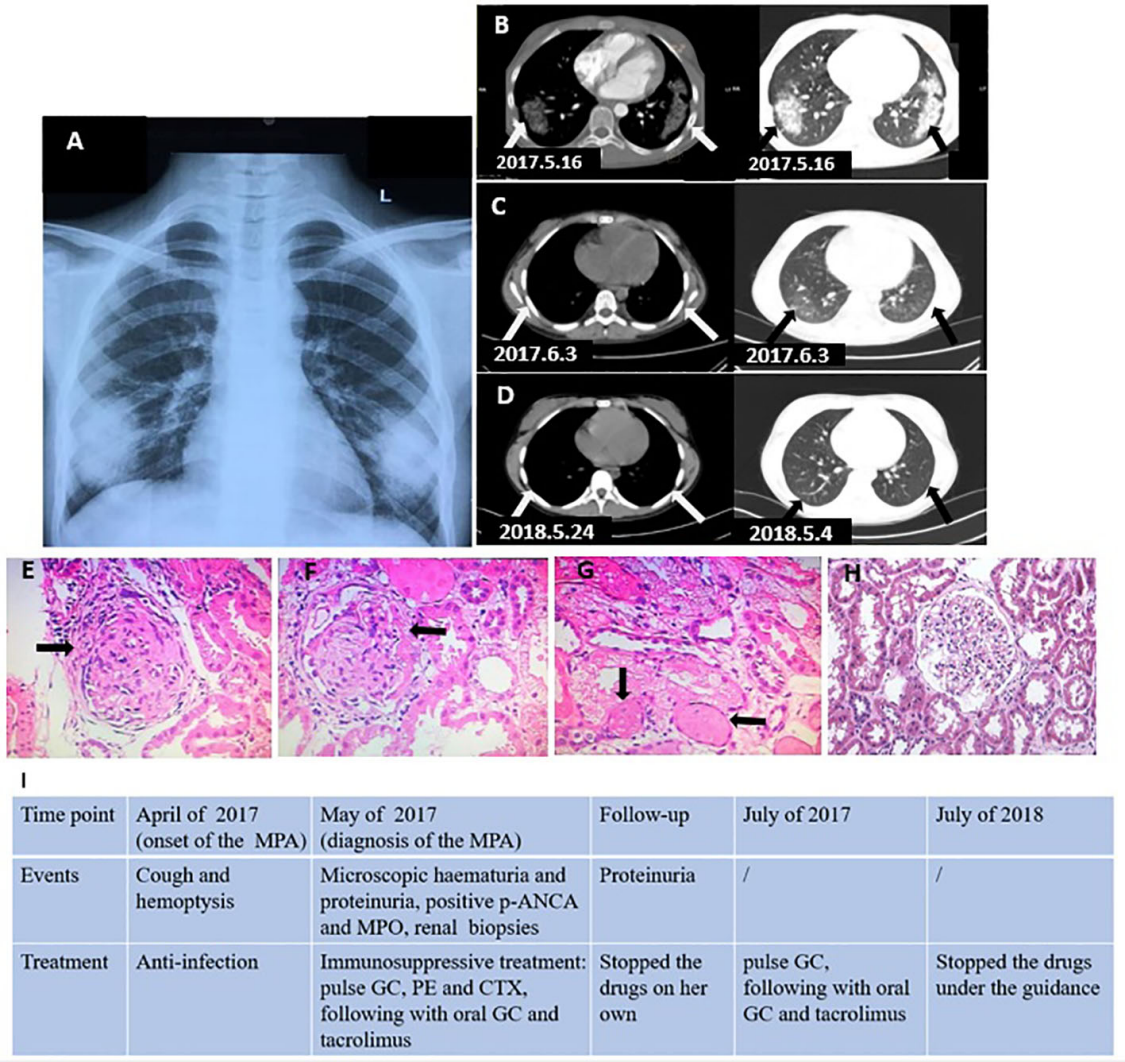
(A) X-ray revealed abnormal density areas (arrows) in the bilateral lower lobe of the lungs (symmetric distribution). (B) Chest CT revealed a large area of shadow (arrows) in the bilateral lower lobe of the lungs (before the treatment). (C) Chest CT revealed the shadows (arrows) in bilateral lungs improved (during the treatment). (D) Chest CT revealed the lesions disappeared (after the treatment). (E, F) Renal biopsies (HE ×40) revealed glomerulonephritic fibrocellular crescents (arrows). (G) Renal biopsies revealed vascular occlusion (arrows). (H) Normal control glomerulus. (I) Clinical timeline. GC, glucocorticoid; CTX, cyclophosphamide; PE, plasma exchange.
